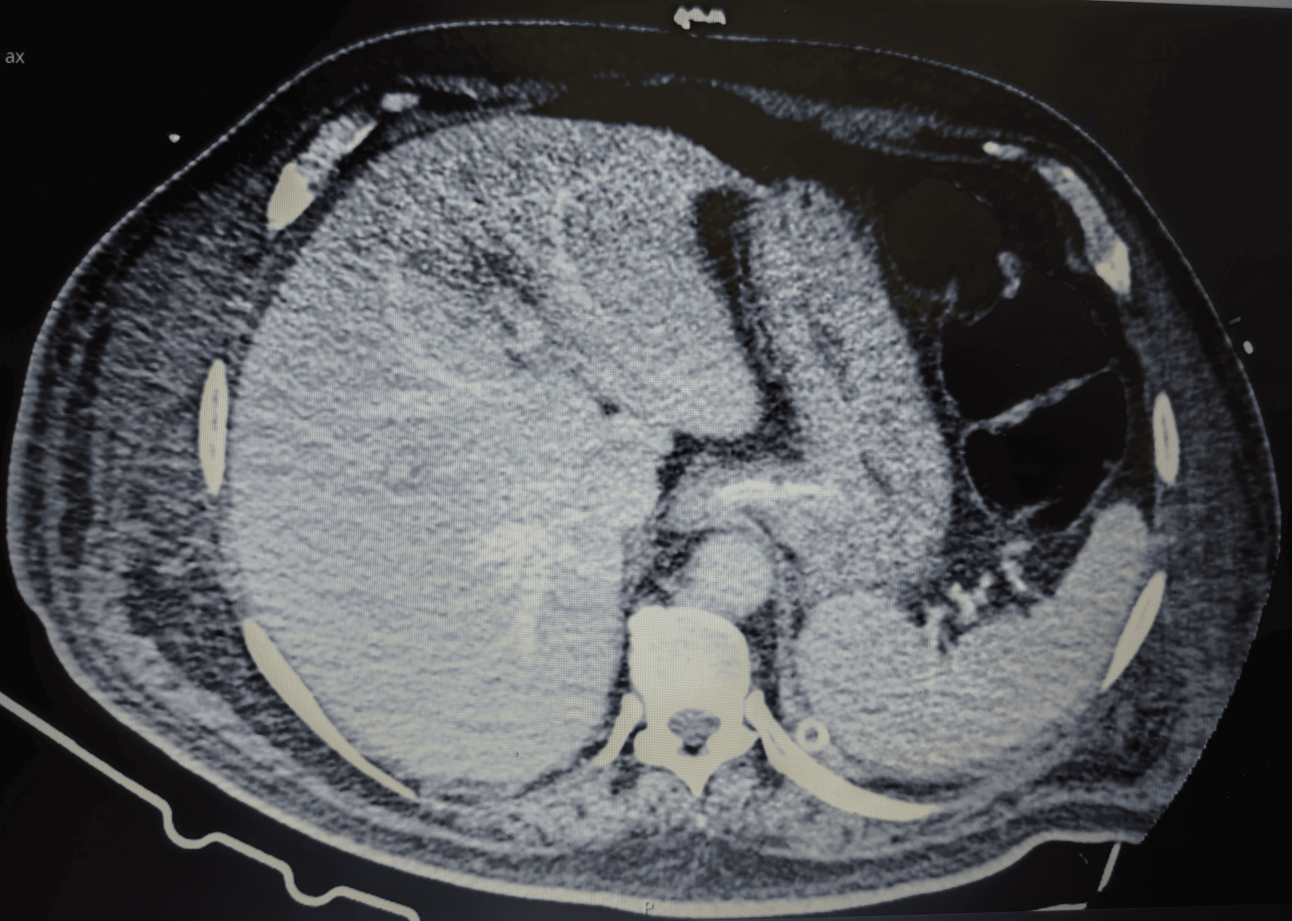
Abdominal computed tomography scan - portal venous phase
